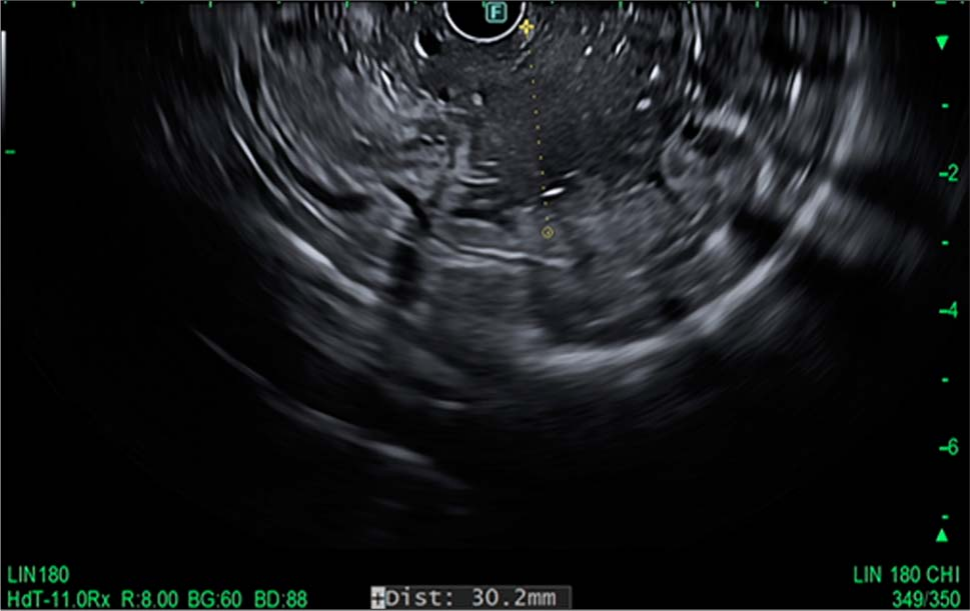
Endoscopic ultrasound findings of gastric wall thickening within the deep mucosa (layer 2).
